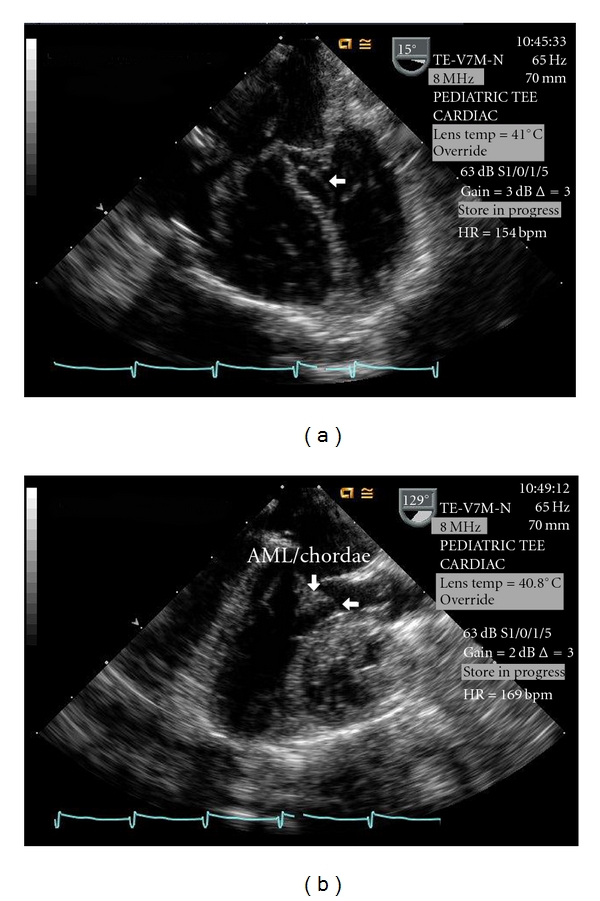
Intraoperative TEE post-VSD repair demonstrating the DVSH (unlabeled arrow) and the systolic narrowing of the LVOT caused by the anterior mitral leaflet/chordal apparatus coming in contact with the DVSH (AML/Chordae). Key: AML/Chordae—anterior mitral leaflet/chordal apparatus, DVSH—dissecting ventricular septal hematoma, LVOT—left ventricular outflow tract, TEE—transesophageal echocardiogram, VSD—ventricular septal defect.
